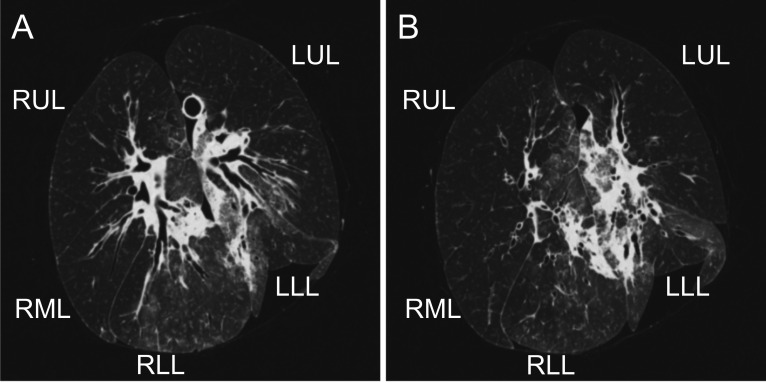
Ex vivo lung CT showing severe infiltration and consolidation in RLL and LLL (A and B). RUL: right upper lobe, RML: right middle lobe, RLL: right lower lobe, LUL: left upper lobe, LLL: left lower lobe
